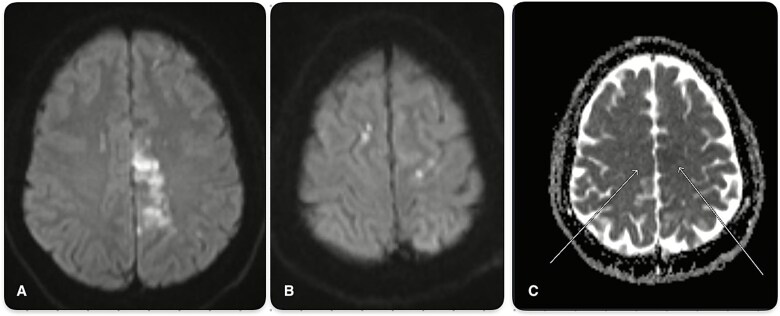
Magnetic resonance imaging (MRI) with diffusion-weighted imaging (DWI b1000) showing (A) acute infraction in the left anterior cerebral artery (ACA) and (B) small foci of acute infarction in bilateral ACA territories. (C) Apparent diffusion coefficient (ADC) scans demonstrate recent infarcts in both ACA territories.
